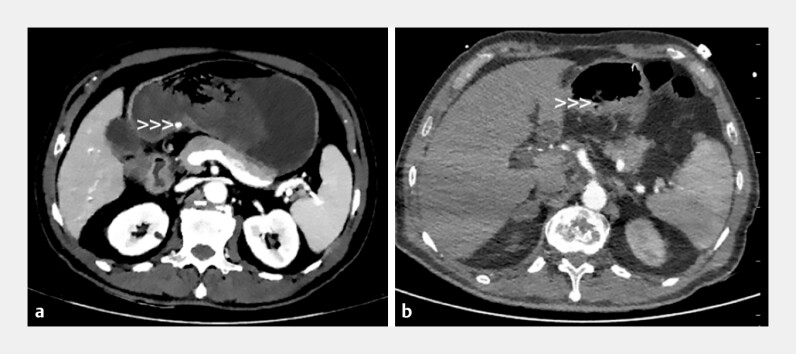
CT images before and 3 days after treatment:
